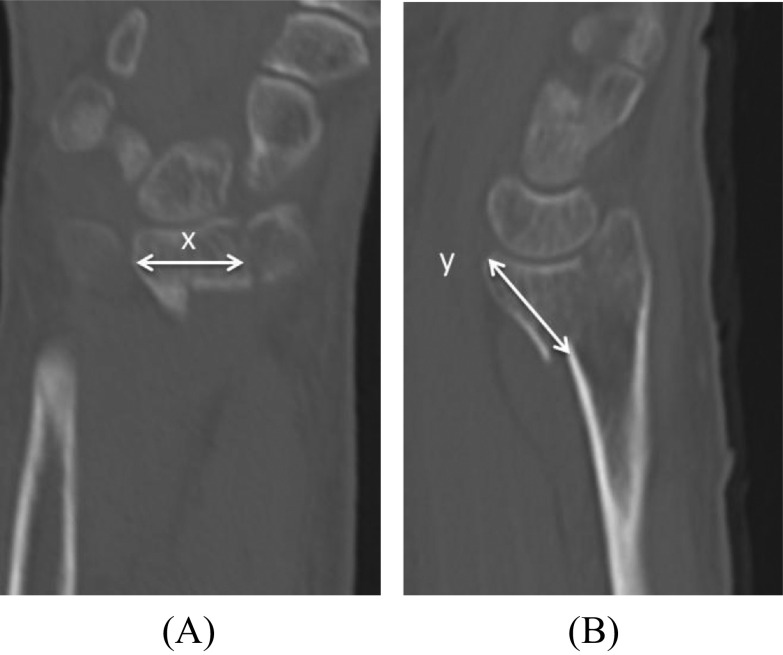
Transverse dimension of the VLF was measured where the distance from radioulnar joint to the radial aspect of fracture line of the VLF was maximum in the CT coronal plane (x) (1A). After the volar tip of the distal radius was identified in the CT axial plane the longitudinal dimension of the VLF was measured at the same point in the CT sagittal plane (y) (1B).
